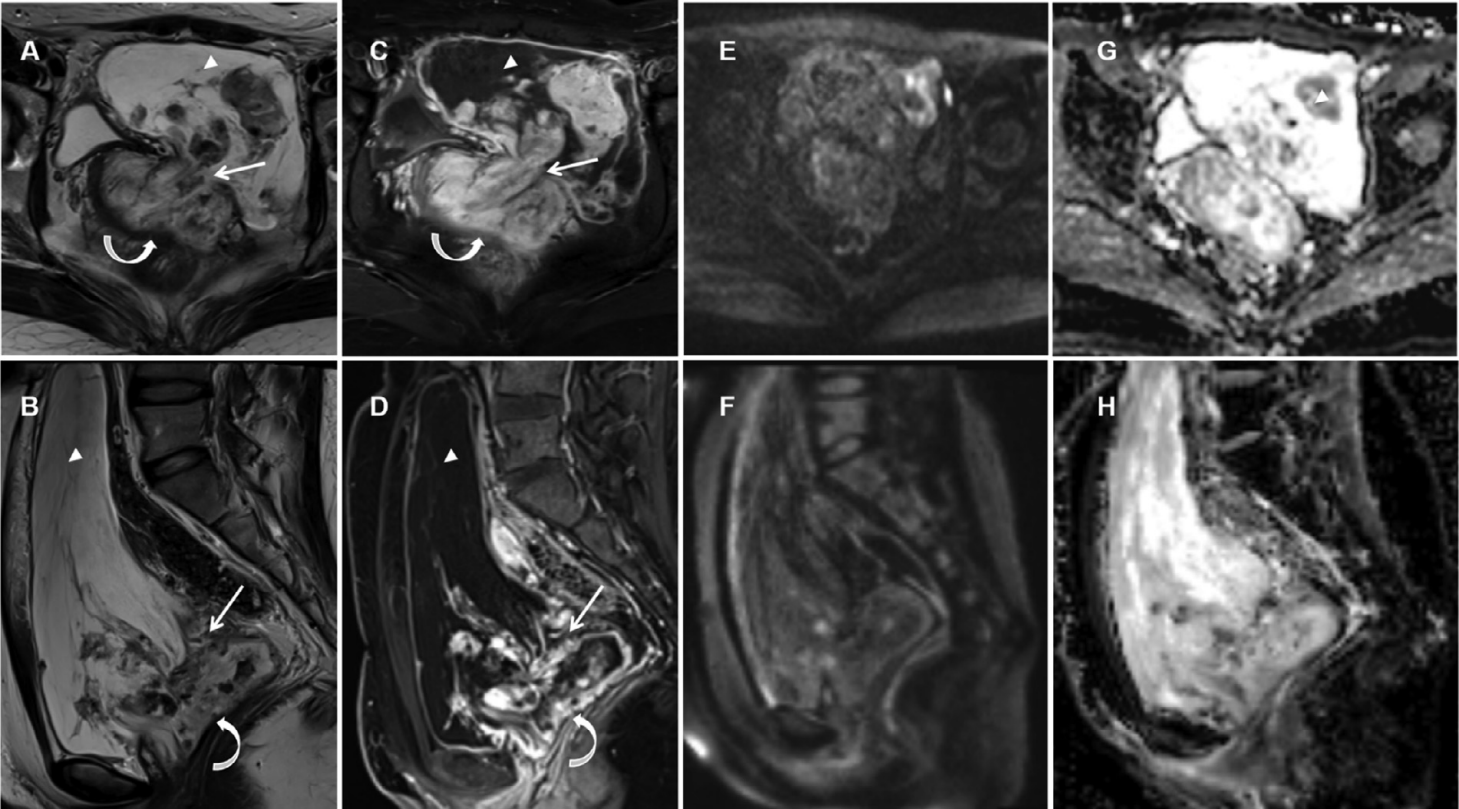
MRI aspect of pelvic tumor and vaginal tumor. (A) Transverse T2WI and (B) sagittal T2WI revealed a pelvic tumor (18 × 7.2 × 11.5 cm, arrowhead) and a vaginal tumor (6.5 × 2.6 × 6.2 cm, curved arrow) were connected at the defect (1.3 × 2.9 cm, arrow) of the vagina. Postcontrast fat-saturated (C) transverse and (D) sagittal T1WI showed the solid components intensely enhanced. (E-H) Diffusion-weighted images showed no restricted diffusion, and the mean apparent diffusion coefficient value was 1.492 × 10^−3^ mm^2^/s (arrowhead).
